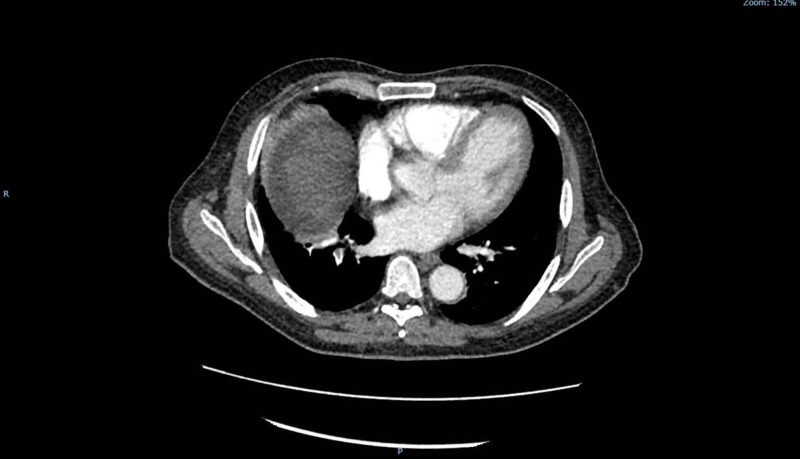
Axial computed tomography of the chest with intravenous contrast showing pulmonary embolism in the right lower lobe of the lung.
